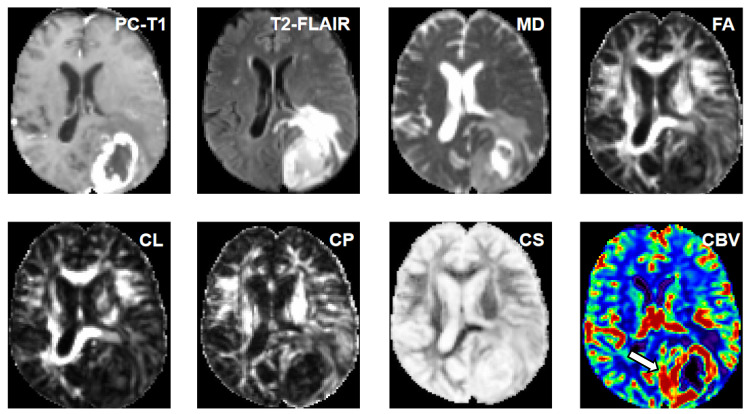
A 65-year-old male patient with a glioblastoma in the left parietal-occipital region. Axial post contrast T1-weighted, and T2-FLAIR images show ring-enhancement and extensive vasogenic edema. DTI derived maps (MD, FA, CL, CP and CS) are shown and CBV map showing high perfusion from CER and IPR of neoplasm indicating neoplastic infiltration (arrow).
